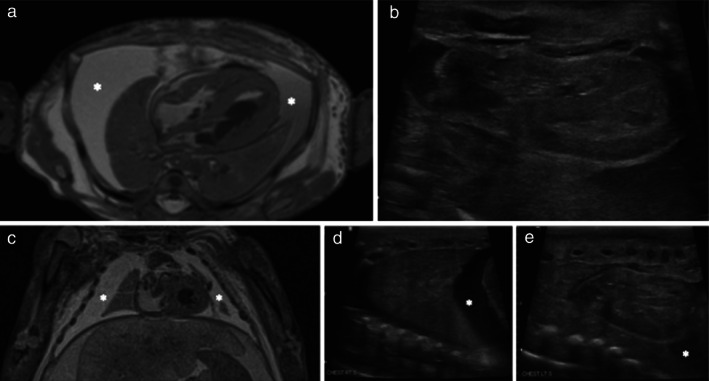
Postmortem imaging in 30‐week fetus with hydrops secondary to underlying cytomegalovirus infection. Neither postmortem ultrasound (PM‐US) nor postmortem magnetic resonance imaging (PM‐MRI) identified any infective process in lungs or presence of cardiomegaly, which was reported at autopsy. (a,b) Axial T2‐weighted PM‐MRI (a) and transverse PM‐US imaging (b) of heart failed to report cardiomegaly, presumably due to more striking appearance of large bilateral pleural effusions (
